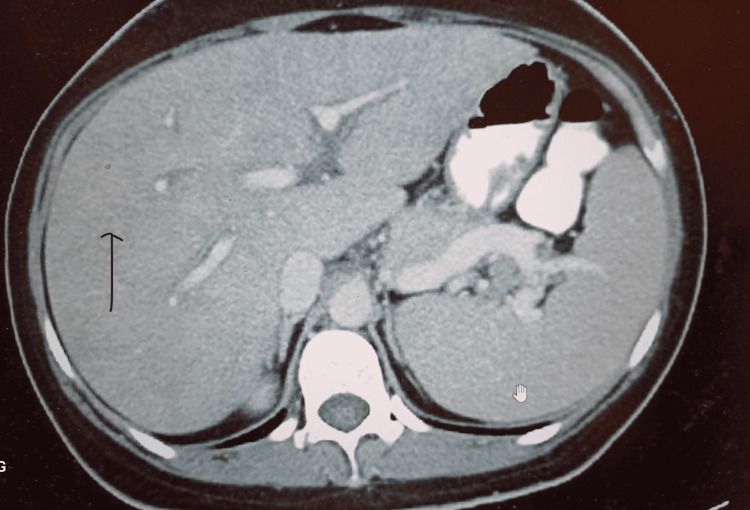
Contrast-enhanced abdominal CT of the patient showing a diffusely enlarged liver without lesions or ascites.
